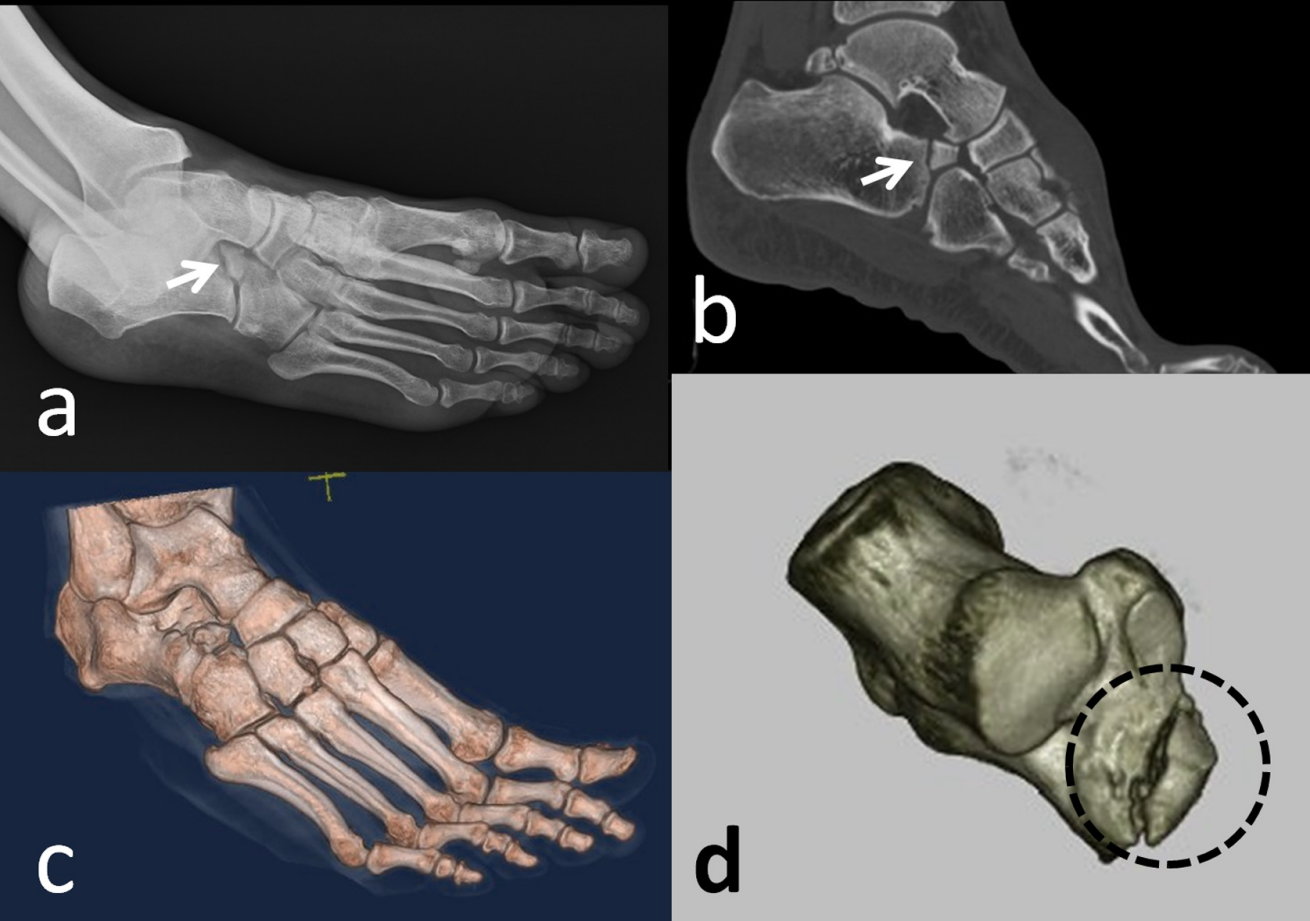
A patient with an anterior process fracture of the calcaneus (a) Foot radiograph; (b) 2D computed tomography (CT) appearance; (c) 3D appearance; (d) 3D appearance - note the sharp edges, communition, and jigsaw puzzle relationship between the fragment and the calcaneus.
